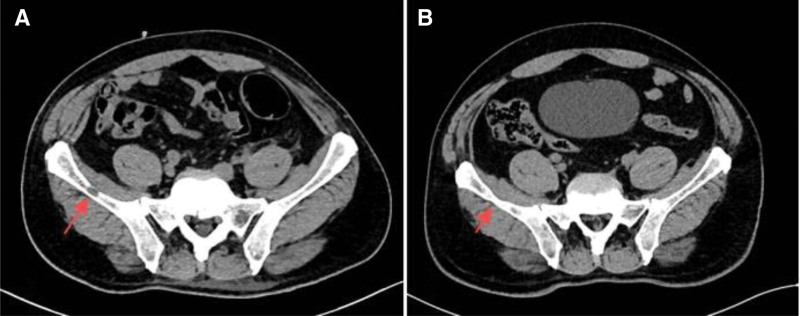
CT appearance of iliac bone metastasis before and after treatment. (A) Follow-up CT after 6 cycles of gemcitabine–cisplatin chemotherapy showing a newly developed osteolytic metastasis in the right iliac bone; (B) follow-up CT in April 2025 postoperatively showing post-treatment changes in the iliac bone metastasis.
